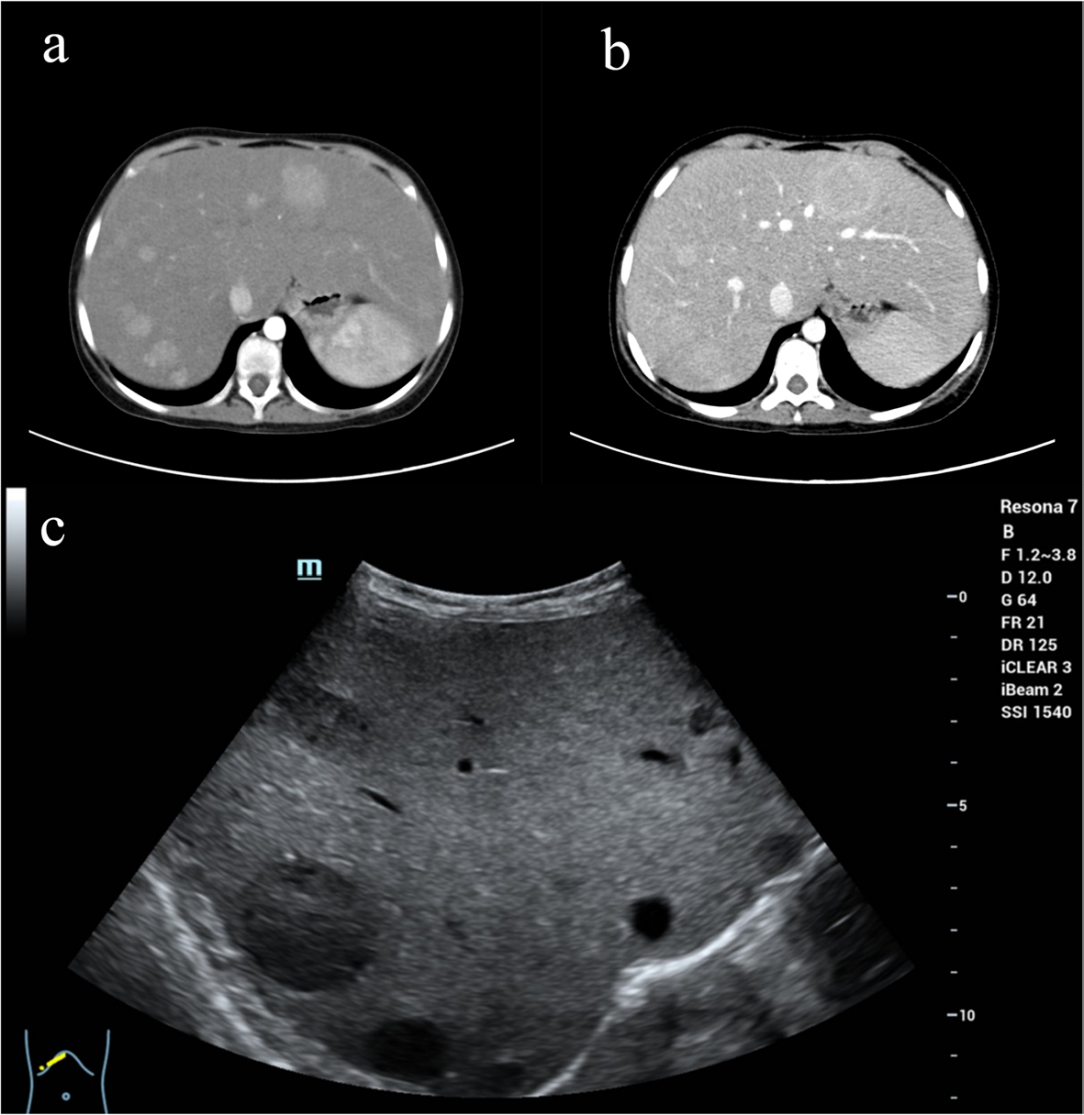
Imaging examination. a The arterial phase of the contrast-enhanced CT image shows multiple nodules and masses in the liver with significant enhancement. b The portal phase of the contrast-enhanced CT image shows that some nodules have mild and persistent enhancement. c B-ultrasonography showed multiple solid nodules in the context of fatty liver
